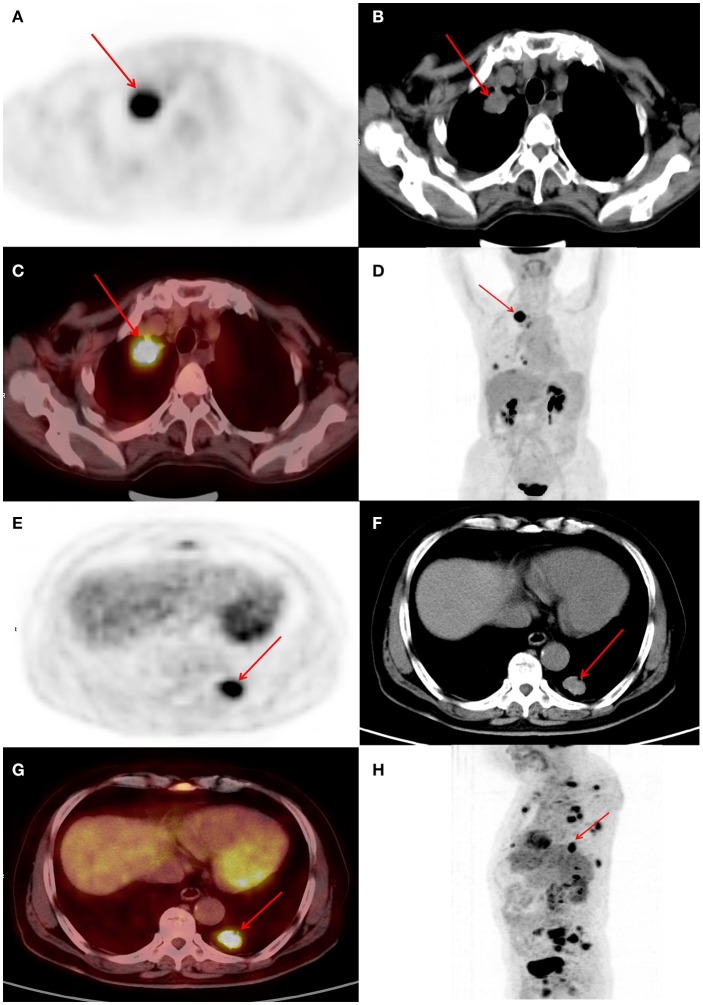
(A–D) Female, 63 years of age. Positron emission tomography/computed tomography (PET/CT) revealing lung adenocarcinoma of the right upper lobe, ~33 × 25 mm, increased fluorodeoxyglucose (FDG) metabolism, maximum standardized uptake value (SUVmax) 6.8, epidermal growth factor (*EGFR*) mutation, and overall survival (OS) of 29 months. (E–H) Male, 72 years of age. Positron emission tomography/computed tomography (PET/CT) revealing lung adenocarcinoma of the posterior basal segment of the left lower lobe, ~27 × 19 mm, increased fluorodeoxyglucose (FDG) metabolism, maximum standardized uptake value (SUVmax) 9.4, epidermal growth factor receptor (*EGFR*) mutation, and OS of 13 months.
